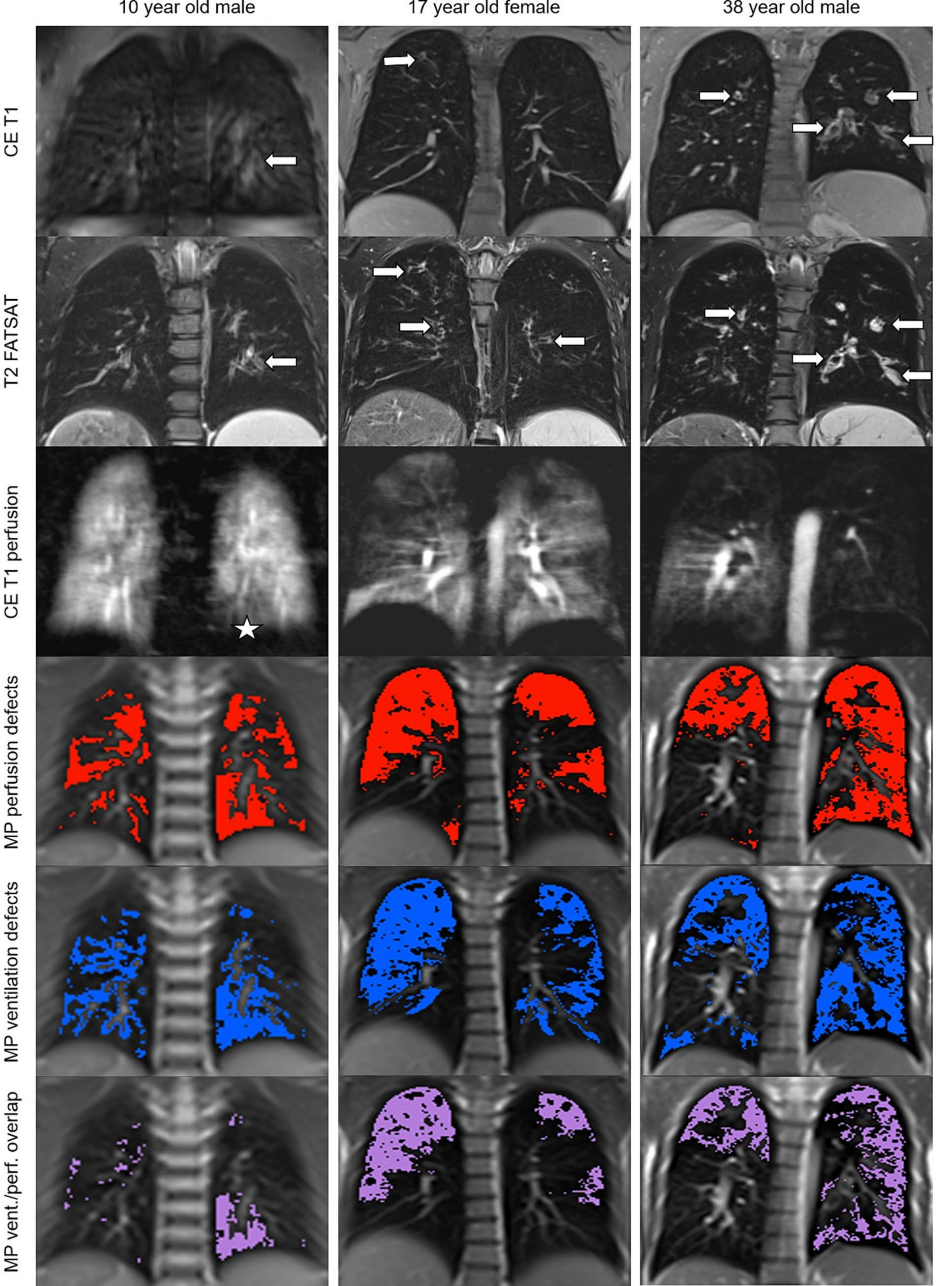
Changes in lung morphology, perfusion and ventilation detected by morpho-functional MRI and MP-MRI in patients with CF. Representative images of a 10 year old school child, a 17 year old adolescent and a 38 year old adult with CF. Contrast-enhanced T1-weighted and fat-saturated T2-weighted MRI shows dilated and/or wall-thickened bronchi (arrows). Contrast-enhanced perfusion MRI and color-coded visualizations of MP-MRI data show areas of decreased lung perfusion and ventilation as well as the overlap between the perfusion and ventilation defects that match with the location of the above morphological changes (asterisk in the youngest patient, obvious in the other two).
